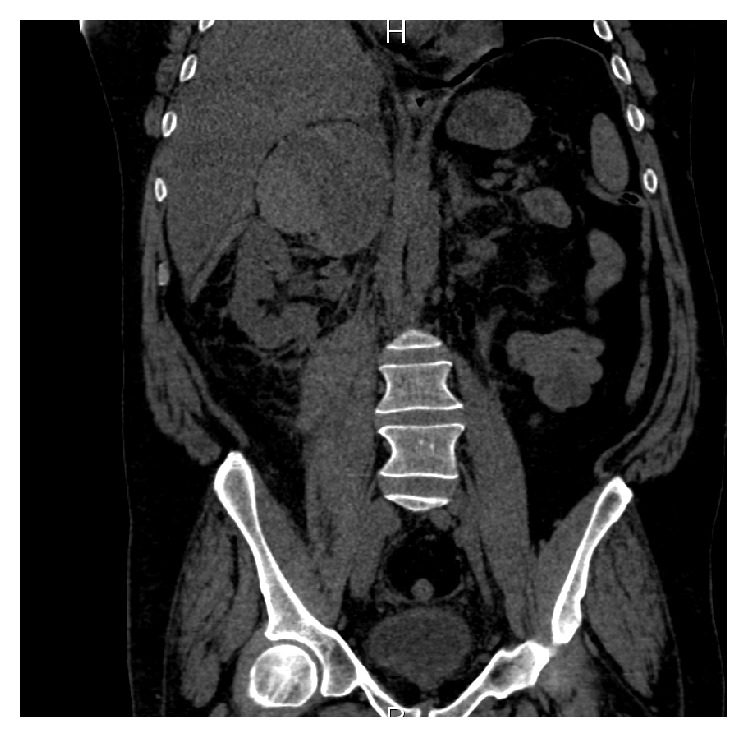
CT scan showing massive right adrenal hemorrhage.
